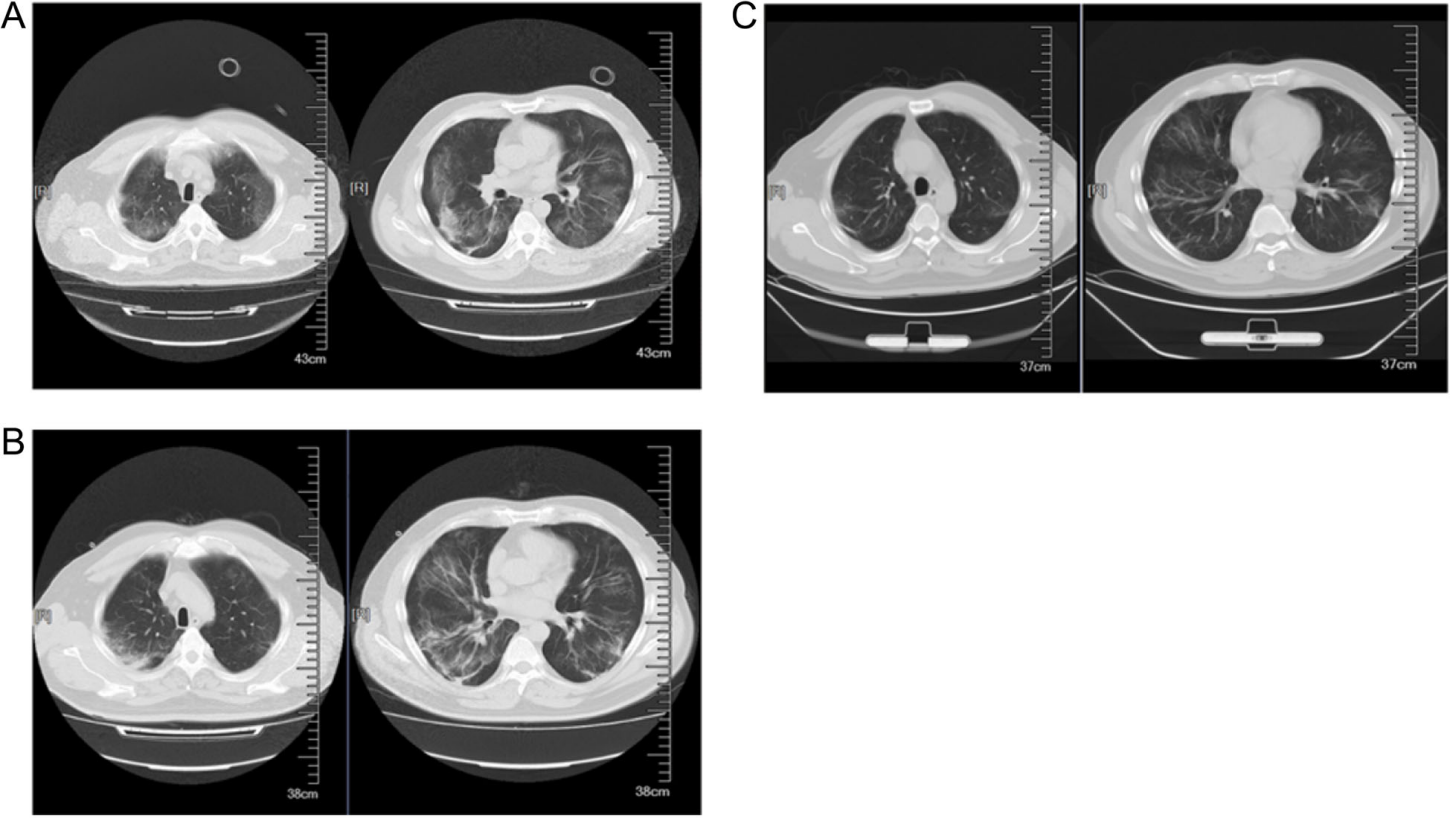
CT scanning results of the lungs. a. CT scan images on 25 January. b. CT scan images on 29 January. c. CT scan images on 12 February. Improvements in abnormalities could be seen
